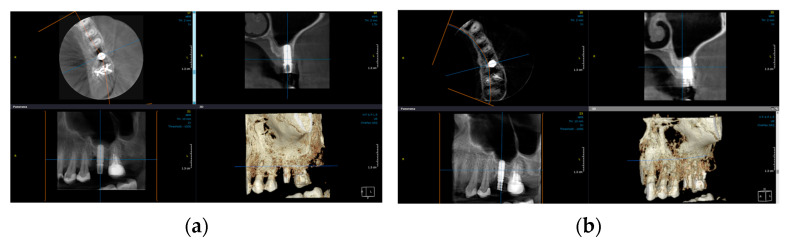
Radiographic imagines representing the marginal bone (MB) changes measured at baseline (a) and six months after surgery (b).
